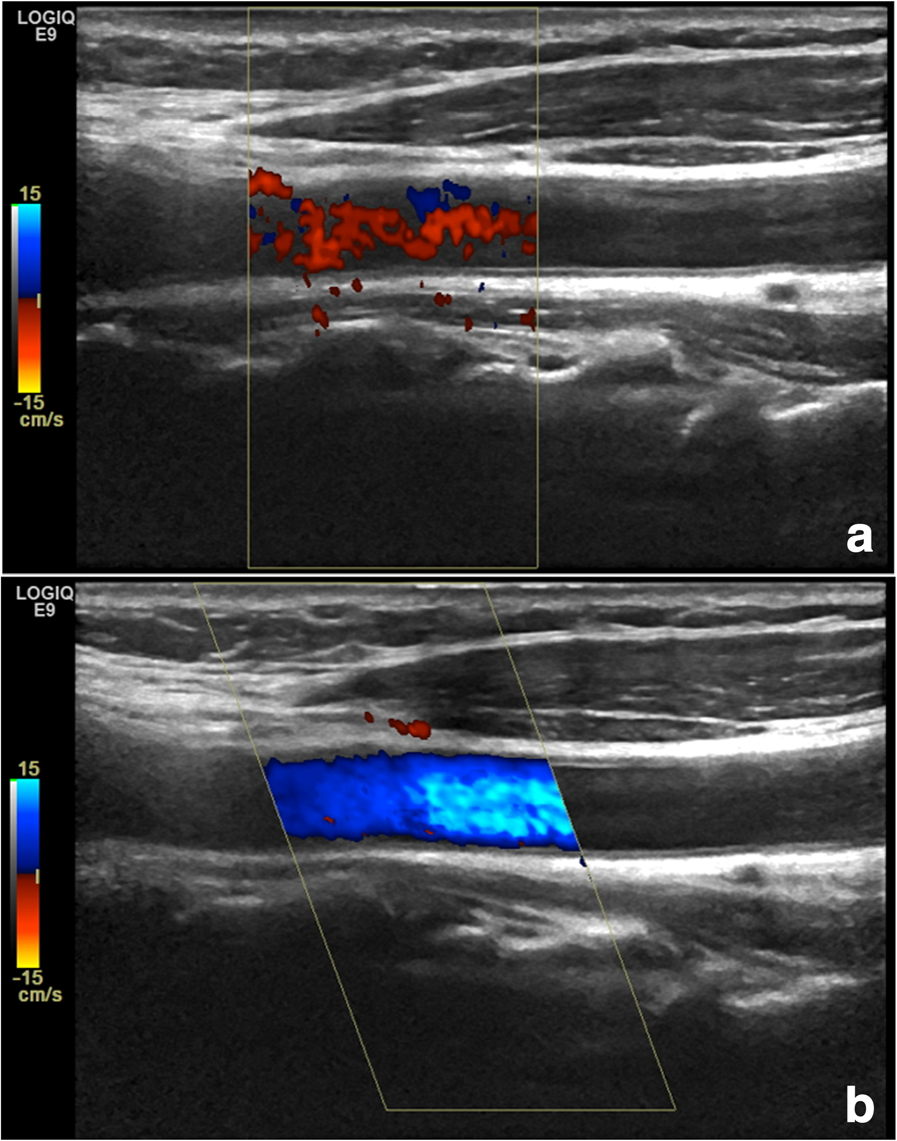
The insonation angle is the angle between the path of the Doppler pulses and the direction of flow in the vessel as indicated by the orientation of the Doppler box. When this angle is 90° (*top*), there will be no frequency shift because cos(90°) = 0. With angle correction in this carotid artery more of the flow becomes detectable (*bottom*)
